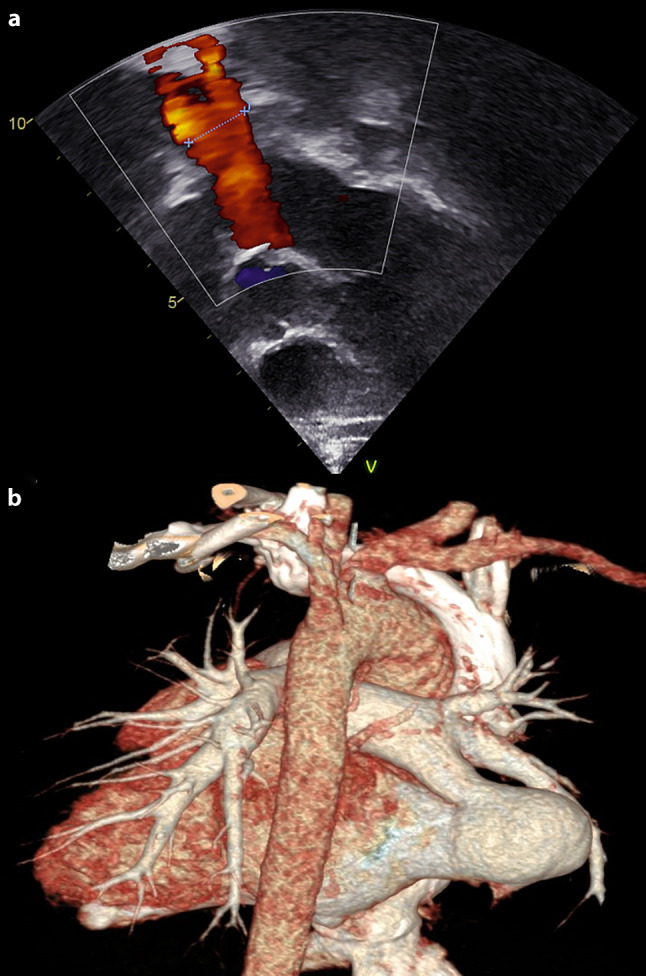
a Colour Doppler study showing massive pulmonary vein flow from the right pulmonary vein. b Volume rendering technique reconstruction of performed computed tomography angiography with surprising finding
